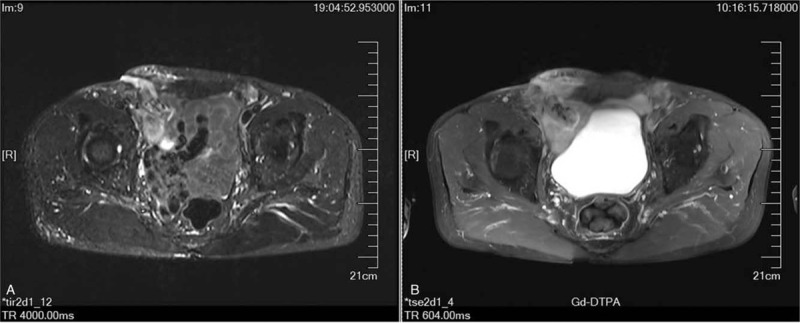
(A) Plain and (B) enhancement magnetic resonance imaging scan of the same plain (different time, different degree of bladder filling).
